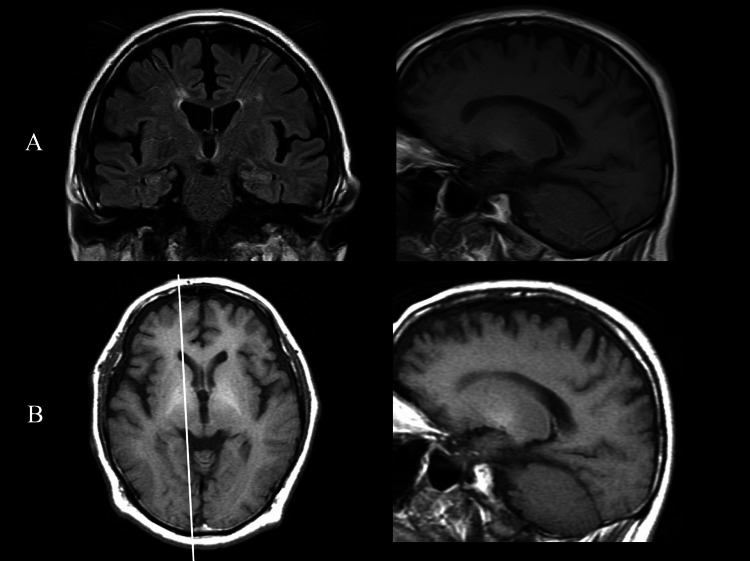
Brain MRI (A) At the initial outpatient visit, coronal FLAIR imaging revealed mild diffuse cerebral atrophy and subtle periventricular ischemic changes. Sagittal T1-weighted imaging showed hyperintensity in the globus pallidus. (B) At hospital admission, axial T1-weighted imaging demonstrated increased signal intensity in the globus pallidus (white arrow), with the corresponding sagittal T1-weighted image confirming the hyperintensity more clearly compared with the previous scan. FLAIR, fluid-attenuated inversion recovery
